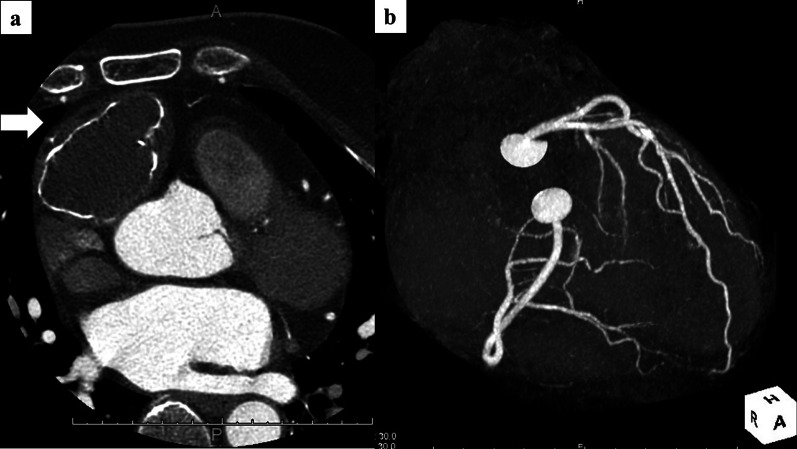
a Chest computed tomography image showing a 50 × 45 × 40 mm mass in the anterior mediastinum with irregular marginal calcification. b Coronary CT shows no coronary aneurysm or feeding artery from the coronary artery
